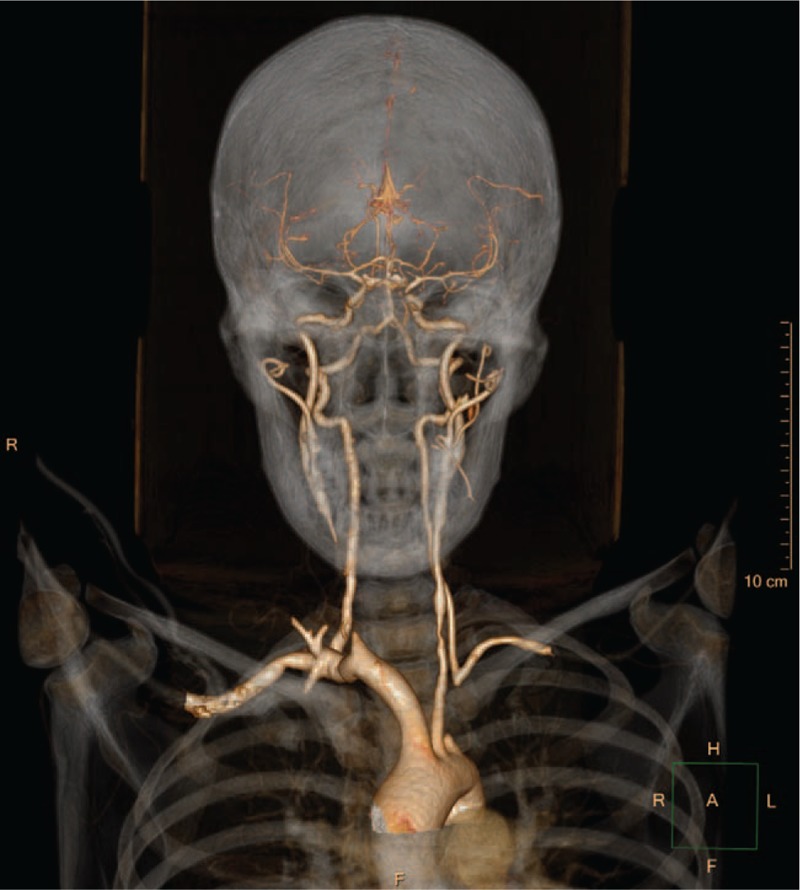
CTA of head and neck artery. CTA showed stenosis in the middle segment of left subclavian artery (LSCA) and mid-distal part of right common carotid artery (RCCA).
